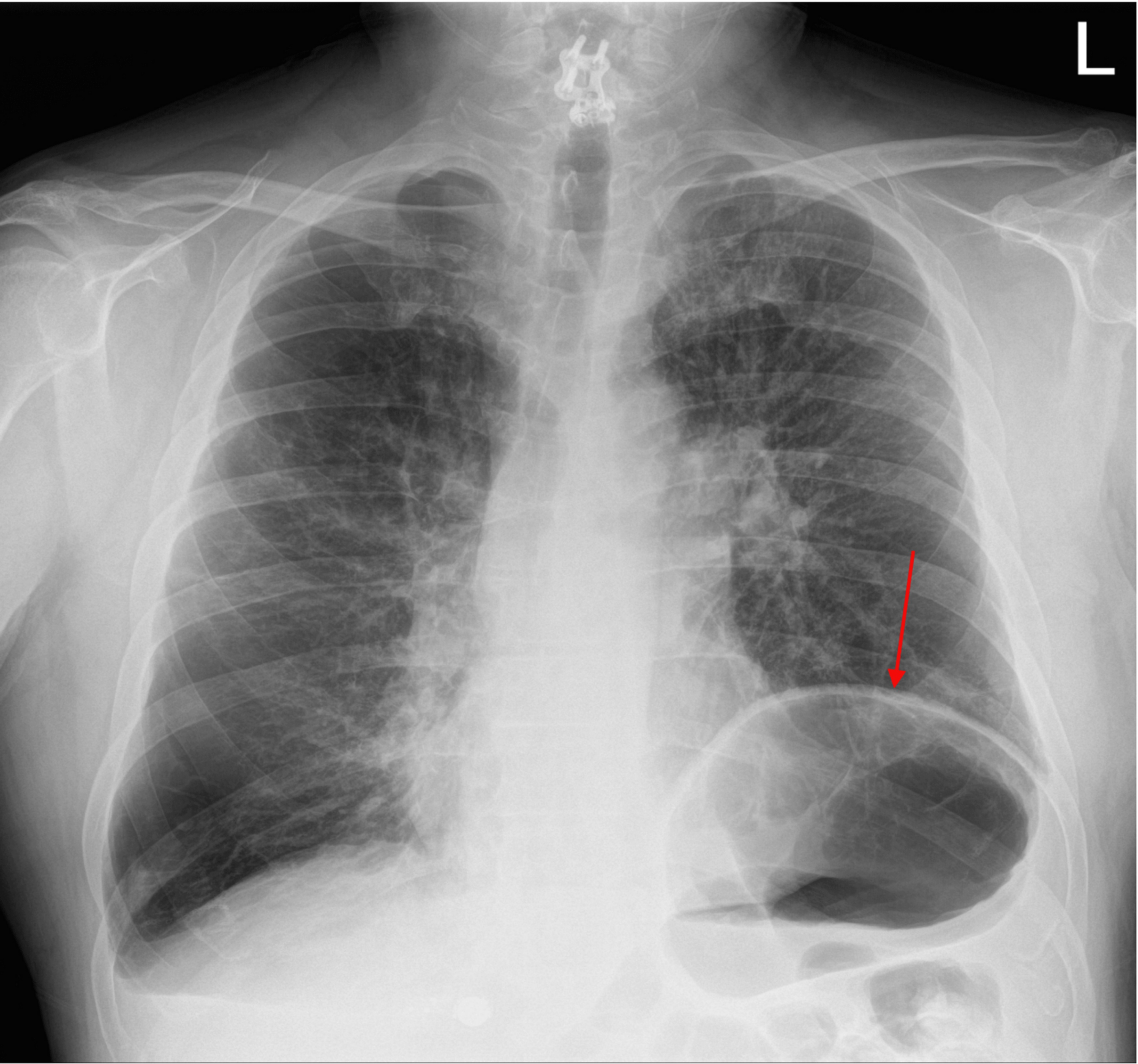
Chest radiograph showing elevated left hemidiaphragm (red arrow), consistent with presentation of dyspnea and history of SPS. SPS: stiff-person syndrome
